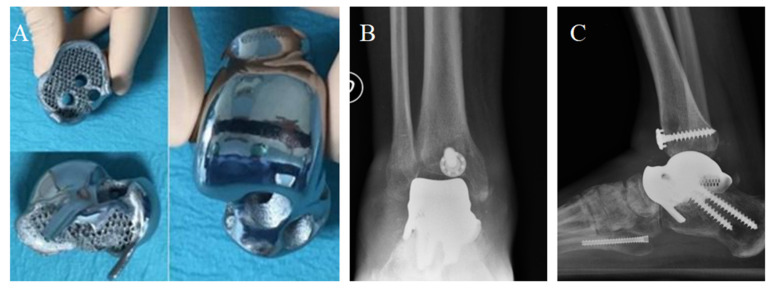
The prosthesis ready to be implanted (A). X-ray of the implant from antero-posterior (B) and latero-lateral (C) projections. Articular space can be observed in figure (B).
